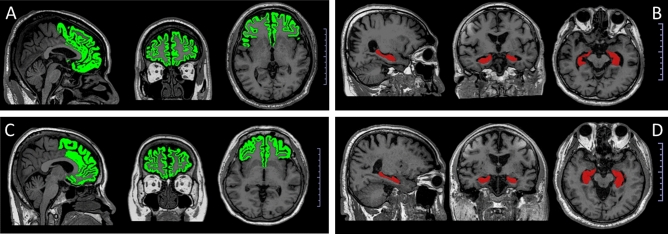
Representation of MRI brain images of participants, the prefrontal cortex identified in green and the hippocampus in red (scale bar, 1 cm × 10 scale); (A) the brain of a 59-year-old man with a MoCA score = 25, total prefrontal cortex percentage = 26.9 and circulating lipocalin level in 2020 = 8.59 ng/ml; (B) the brain of a 65-year-old man with a MoCA score = 27, total hippocampus percentage = 0.6 and circulating lipocalin level in 2020 = 21.15 ng/ml; (C) the brain of a 60-year-old man with a MoCA score = 24, total prefrontal cortex percentage = 23.4 and circulating lipocalin level in 2020 = 21.98 ng/ml; (D) the brain of a 60-year-old man with a MoCA score = 25, total hippocampus percentage = 0.49, and circulating lipocalin level in 2020 = 13.07 ng/ml.
